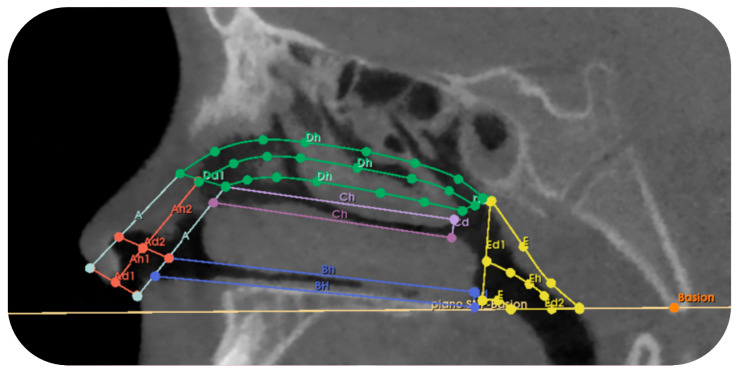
CBCT visualization of the subdivision of the nasal cavity with the respective references and measurements for the study of airflow: diameter (Ad1) and height (Ah1) of solid A1 and the height (Ah2) of solid A2; length (Bh) and diameter (Bd) of solid B; length (Ch) and diameter (Cd); length (Dh) and diameter (Dd2) of solid D; diameter 1 (Ed1), diameter 2 (Ed2), and height (Eh) of solid E.
